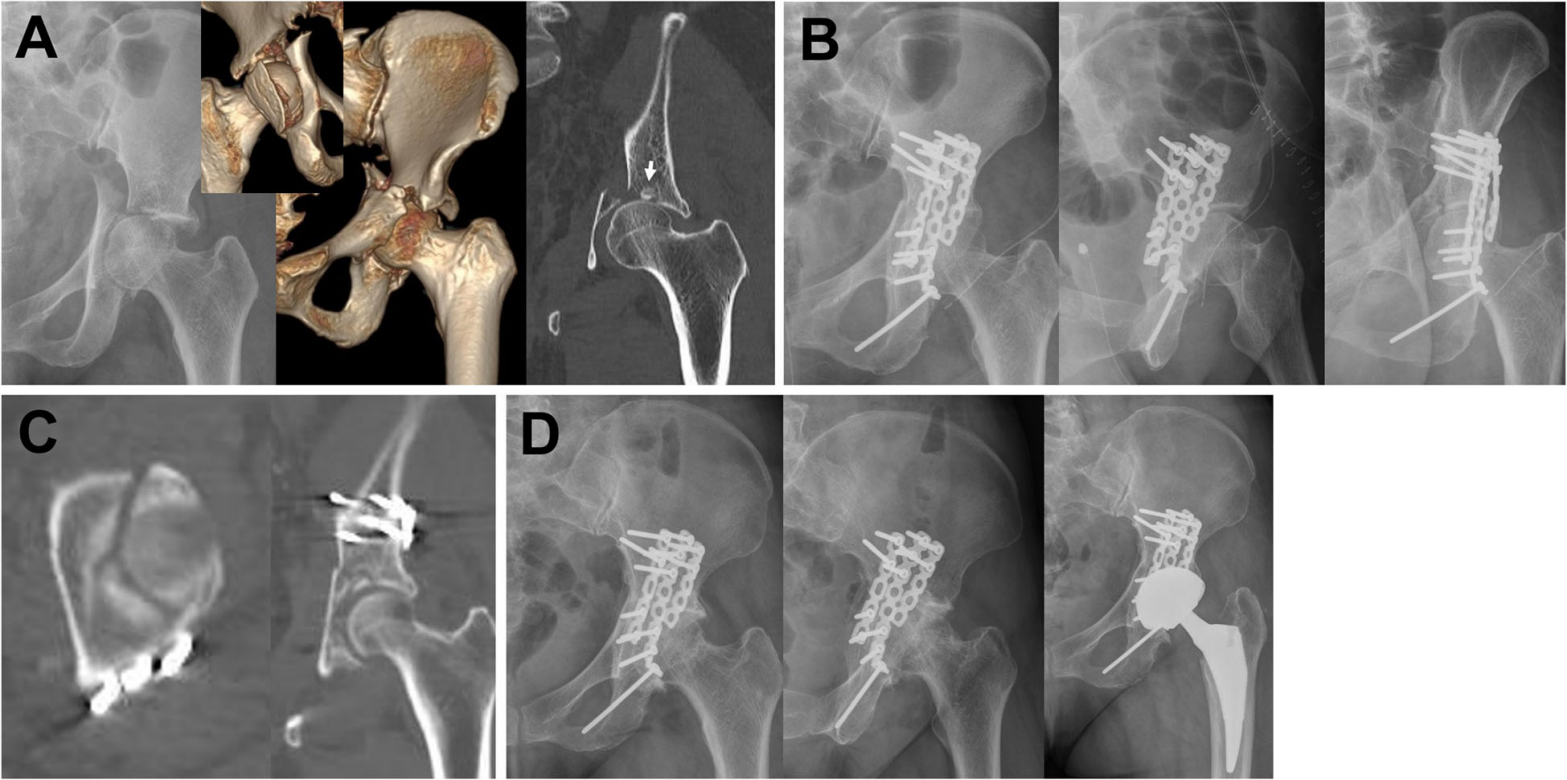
A 47-year old woman involved in a traffic accident. a Immediate radiograph and three-dimensional computed tomography (CT) images show transverse and posterior wall fractures of acetabulum. Dome impaction (arrow) was observed in coronal view of CT scan. b Postoperative radiographs revealed that satisfactory reduction and fixation were achieved. c However, axial and coronal CT views show a residual gap > 3 mm and poor reduction and fixation of the dome fragment. d Osteoarthritis developed and total hip arthroplasty was performed at 6 months after surgery
